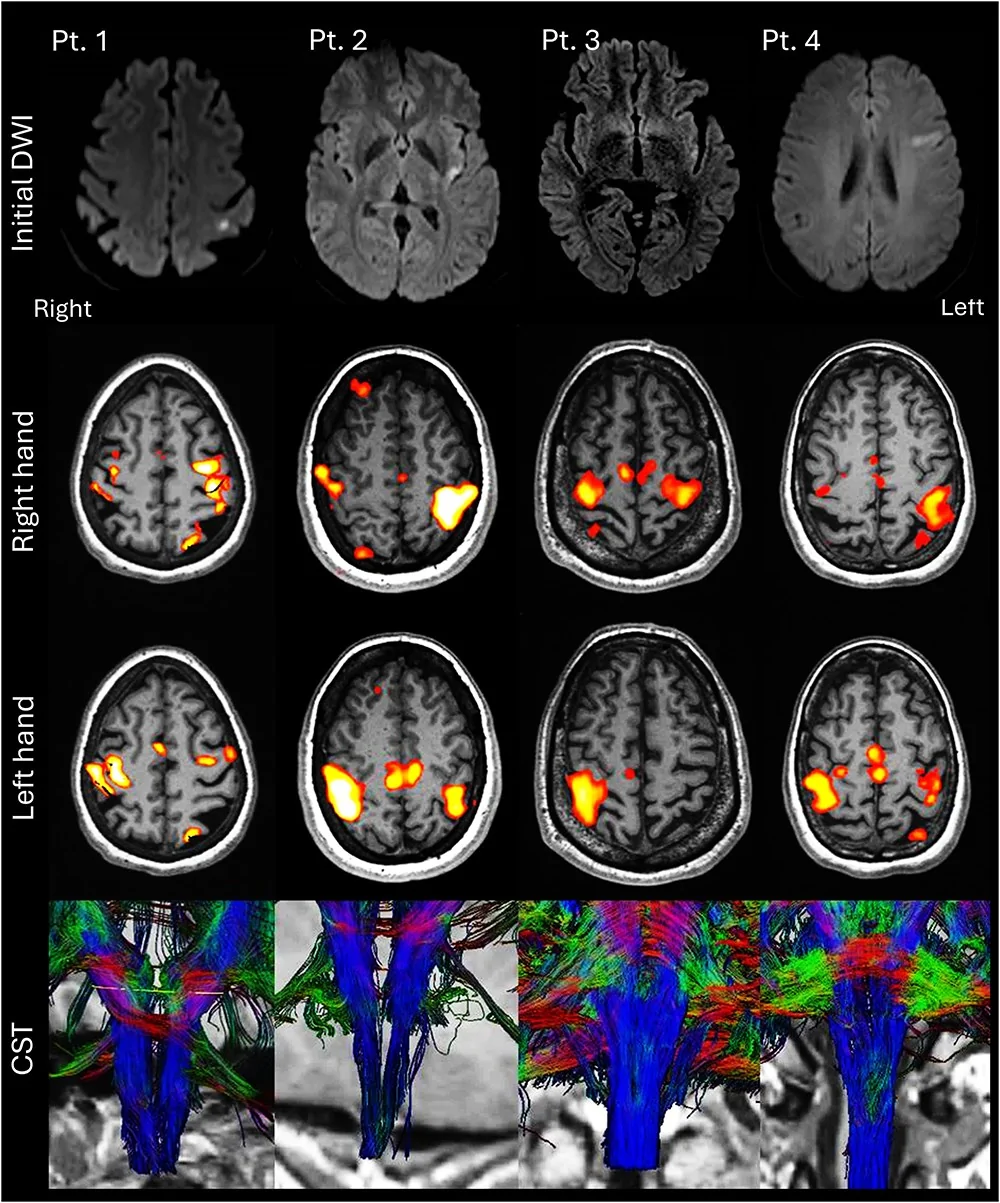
(Results, single-centre experience): Neuroimaging findings in the 4 patients with ipsilateral hemiparesis. Top row: DWI demonstrating acute ischaemic lesions in the left MCA territory in all 4 patients. Middle rows: Functional MRI (BOLD) activation maps during right- and left-hand finger tapping tasks showing predominantly bilateral cortical motor activation, including activation of the ipsilateral hemisphere relative to the moving limb. Bottom row: DTI tractography demonstrating physiological decussation of the CSTs in all patients. Abbreviations: BOLD = blood oxygen level–dependent; CST = corticospinal tract; DTI = diffusion tensor imaging.
